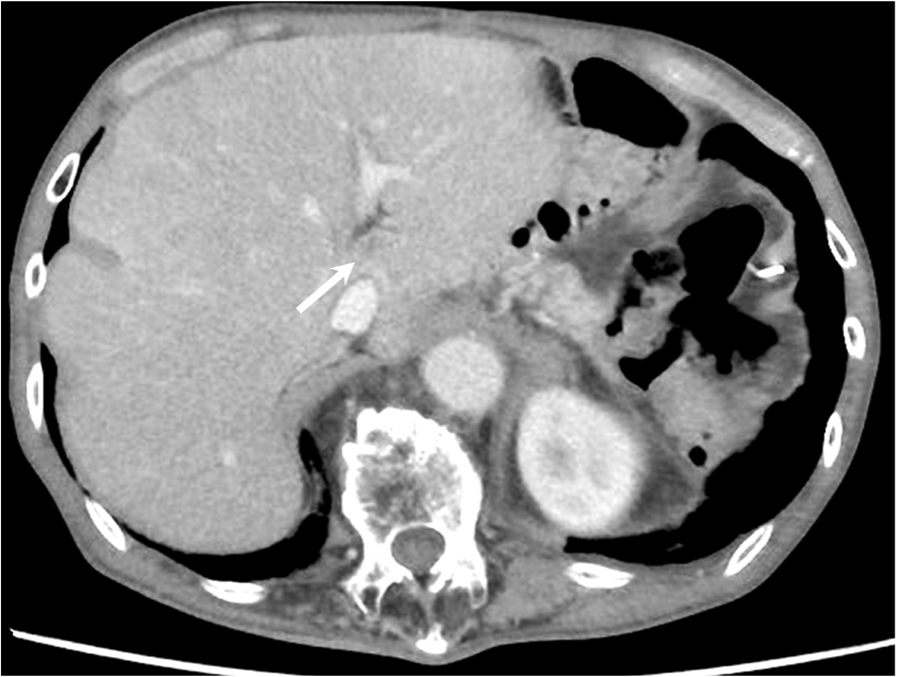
Abdominal computed tomography (CT) findings after eight cycles of nivolumab. Abdominal enhanced-contrast CT revealed a further reduction (by 82.6%) in liver metastases (arrow)
